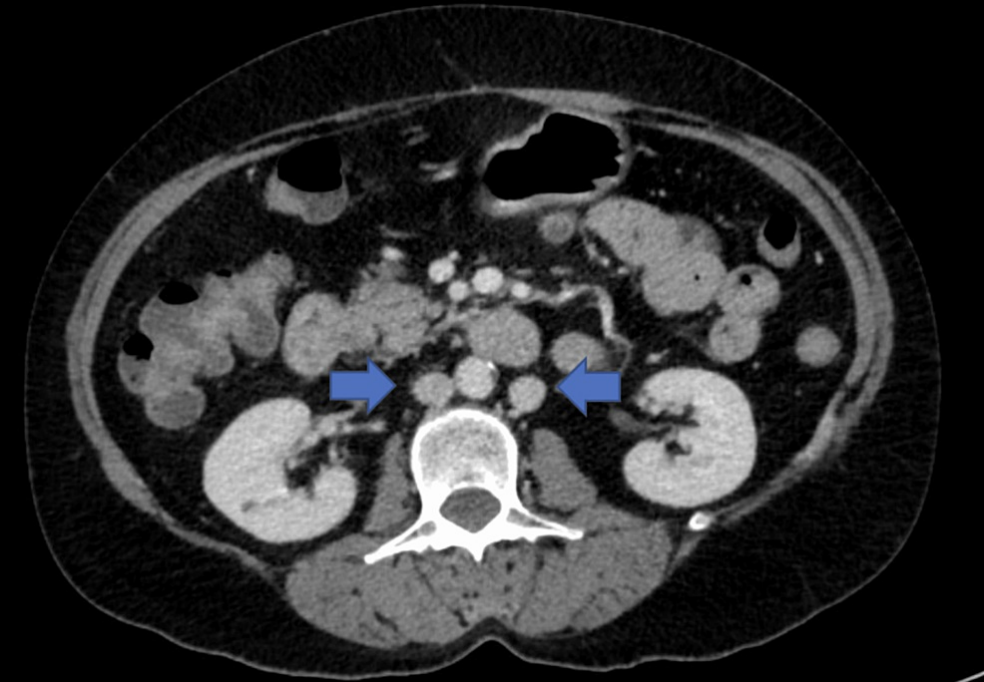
CT abdomen and pelvis, axial reconstruction, shows duplicated infrarenal inferior vena cava (blue arrows)
